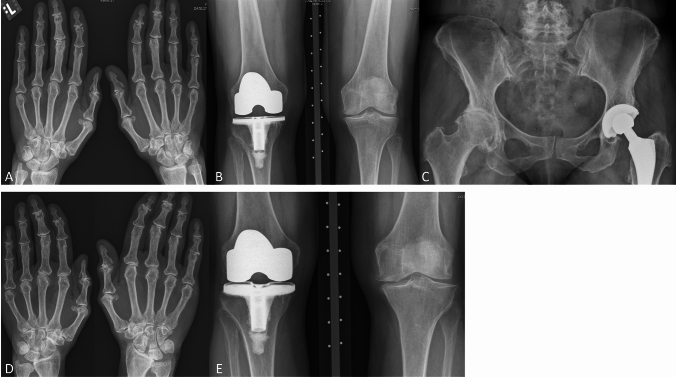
Radiographic examination of the hands, knees, and hips with follow-up. The first detailed radiographic examination of the joint complaints occurred in 2010 (A, B, C), with a follow-up detailed examination in 2016 (D, E). A Dorsovolar radiograph of the hands. Osteoarthritis of the hands, predominantly in the interphalangeal joints but also in other joints such as the MCP joints. B Posteroanterior radiograph of the knees. Osteoarthritis in the left knee, total knee replacement in the right knee. C Anteroposterior radiograph of the pelvis. Severe osteoarthritis in the right hip, total hip replacement on the left side. D Follow-up dorsovolar radiograph of the hands. Progression of the osteoarthritis in the hands, predominantly in the interphalangeal and MCP joints but also in other joints. E Follow-up posteroanterior radiograph of the knees. Progression of the osteoarthritis in the left knee
